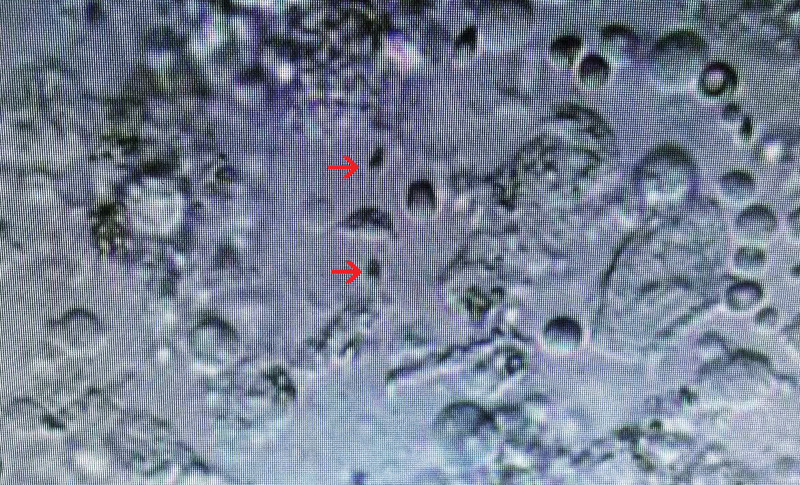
Testicular epididymal sperm aspiration was performed under the guidance of ultrasound. Motile sperms (indicated by red arrows) were obtained for intracytoplasmic sperm injection.
